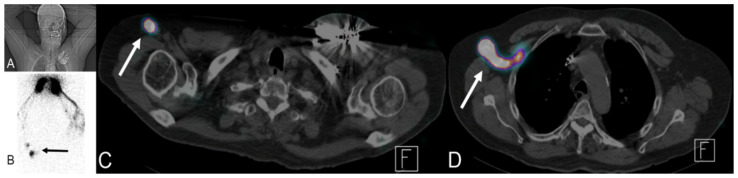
A 76-year-old male patient with a history of tongue base cancer status post-chemoradiation and left upper extremity lympho-venous bypass due to swelling of his left arm. Lymphoscintigraphy was performed by injection of sulfur colloid (radiotracer) into both hands. (A) The patient position. (B) Right anterior scintigraphy position with accumulation of tracer in both hands and tracer uptake in the right arm (black arrow). (C,D) Fused SPECT/CT with transit of tracer via proximal arm LN (white arrow in (C)) to reach lymph nodes at right axilla (white arrow (D)) with absence of any tracer uptake in the whole left upper extremity, confirming normal lymphatic drainage of the right arm with lymphedema of the left arm.
